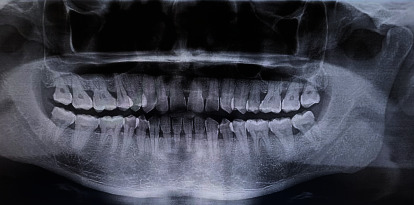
OPG demonstrating an ill-defined periapical pathology with #31.
